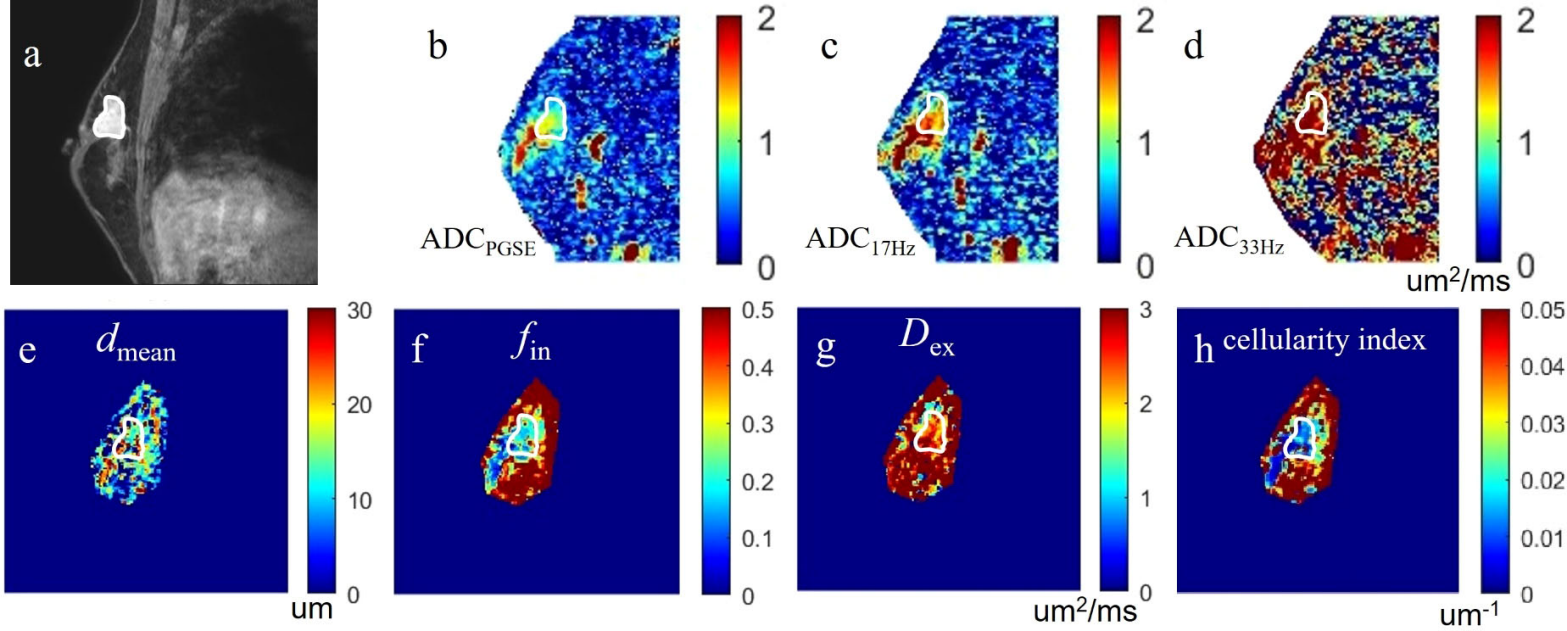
a case of invasive carcinoma in the right breast: (A) the DCE-MRI image as reference; (B-D) the ADC_PGSE,_ ADC_17Hz_ and ADC_33Hz_ images with values for the lesion of 1.021, 1.409, and 2.018 um^2^/ms, respectively; (E-H) the *d*
